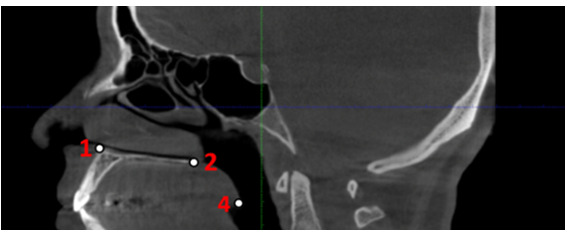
In this analytical cross-sectional study, by random sampling, pre-treatment CBCT
